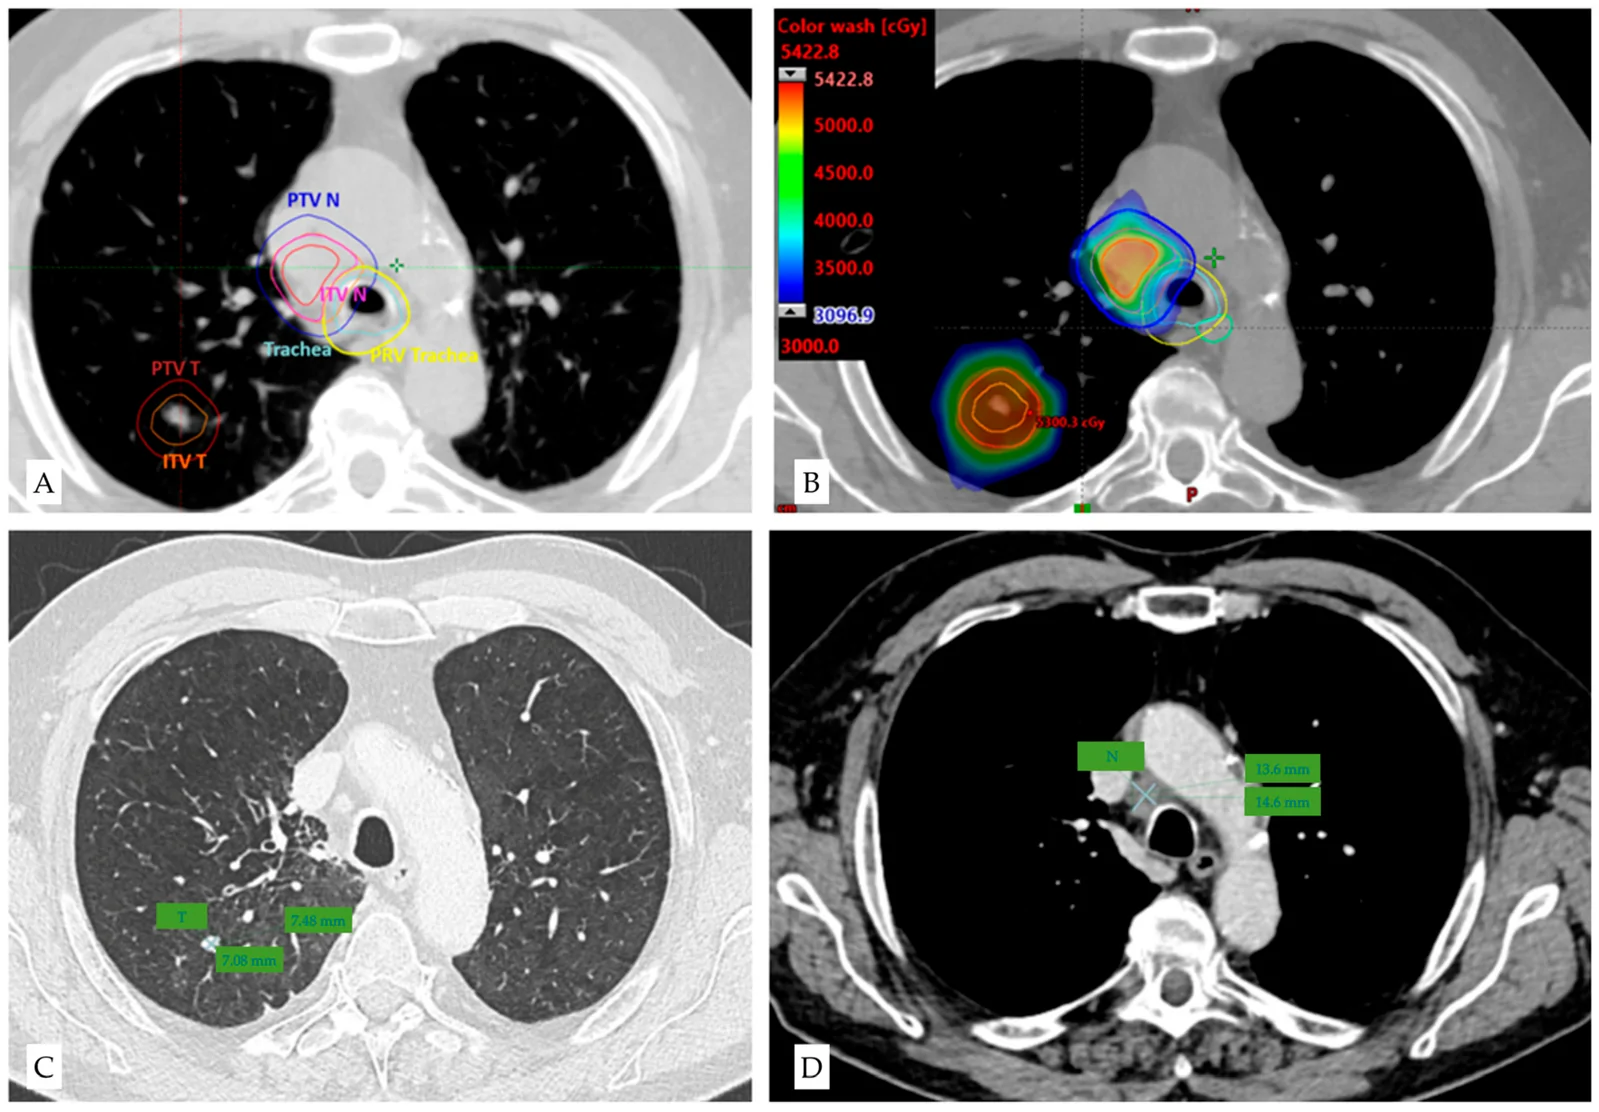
(A) Right upper lobe, unresectable LA-NSCLC, demonstrated on non-contrast four-dimensional radiotherapy planning CT. PTV N, planning tumor volume of involved node; ITV N, internal target volume (ITV) of involved node; PTV T, PTV of primary tumor; ITV T, ITV of primary tumor. (B) Treatment plan with dose superimposed. Stereotactic ablative body radiotherapy (SABR) was the technique of choice. Through the simultaneous integrated boost (SIB) technique, 30 Gy, 40 Gy, and 50 Gy were prescribed to PTV N, ITV N, PTV T and a subvolume of ITV N, respectively. (C,D) A diagnostic CT 9 months after SBRT demonstrated the marked partial response of the primary tumor and involved node.
